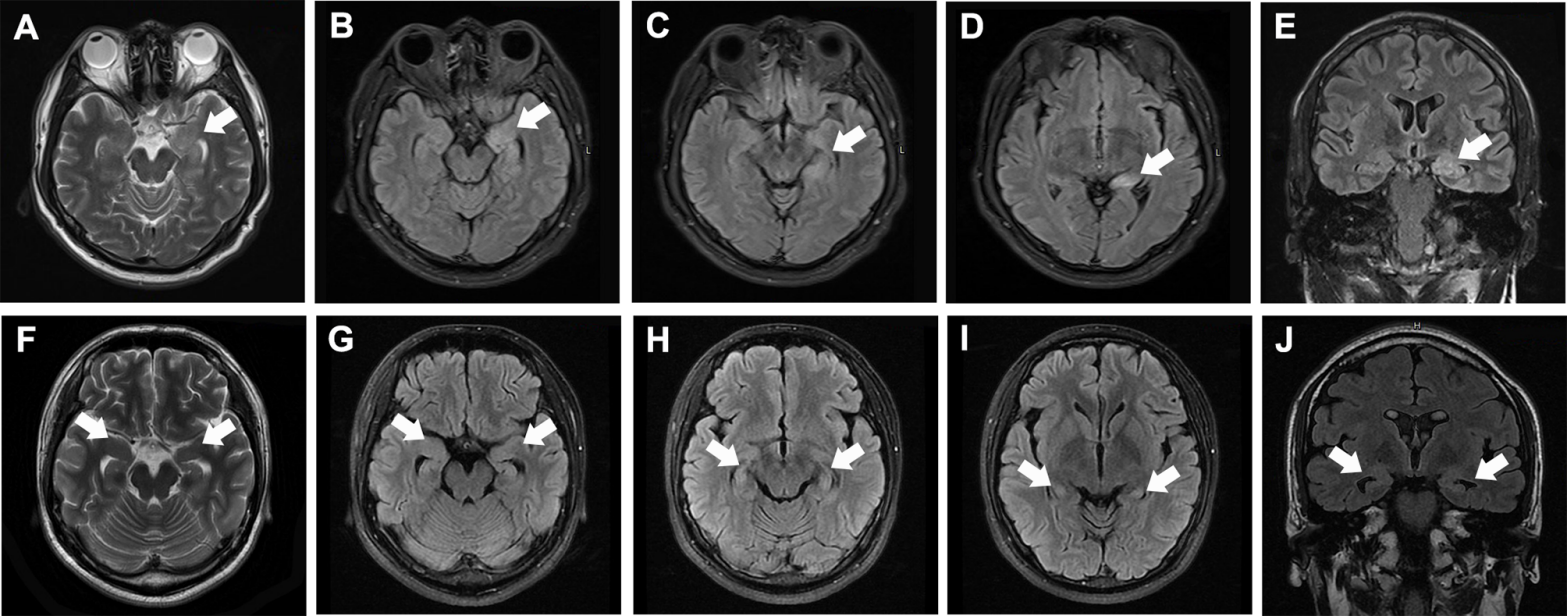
Typical MRI of anti-LGI1 encephalitis patients. (A–E) MRI of a patient at 1 month after disease-onset, showing high T2 (A) and T2/FLAIR [(B–D axial, (E) coronal] signals in the left hippocampus (white arrows). (F–J) MRI of a patient at 6 months after disease-onset, showing moderately elevated T2 (A) and T2/FLAIR [(B–D) axial, (E) coronal] signals in both hippocampi (white arrows) as well as bilateral hippocampal atrophy.
